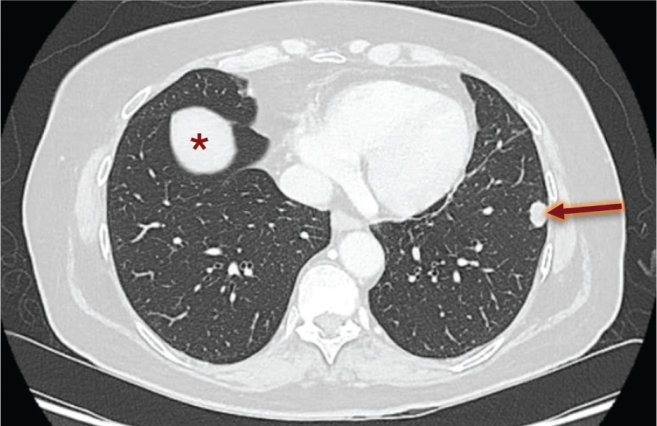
Single lung metastasis before wedge resection (indicated by an arrow). *Axial liver cross-section showing on CT-scan of thorax; no metastatic lesion.
